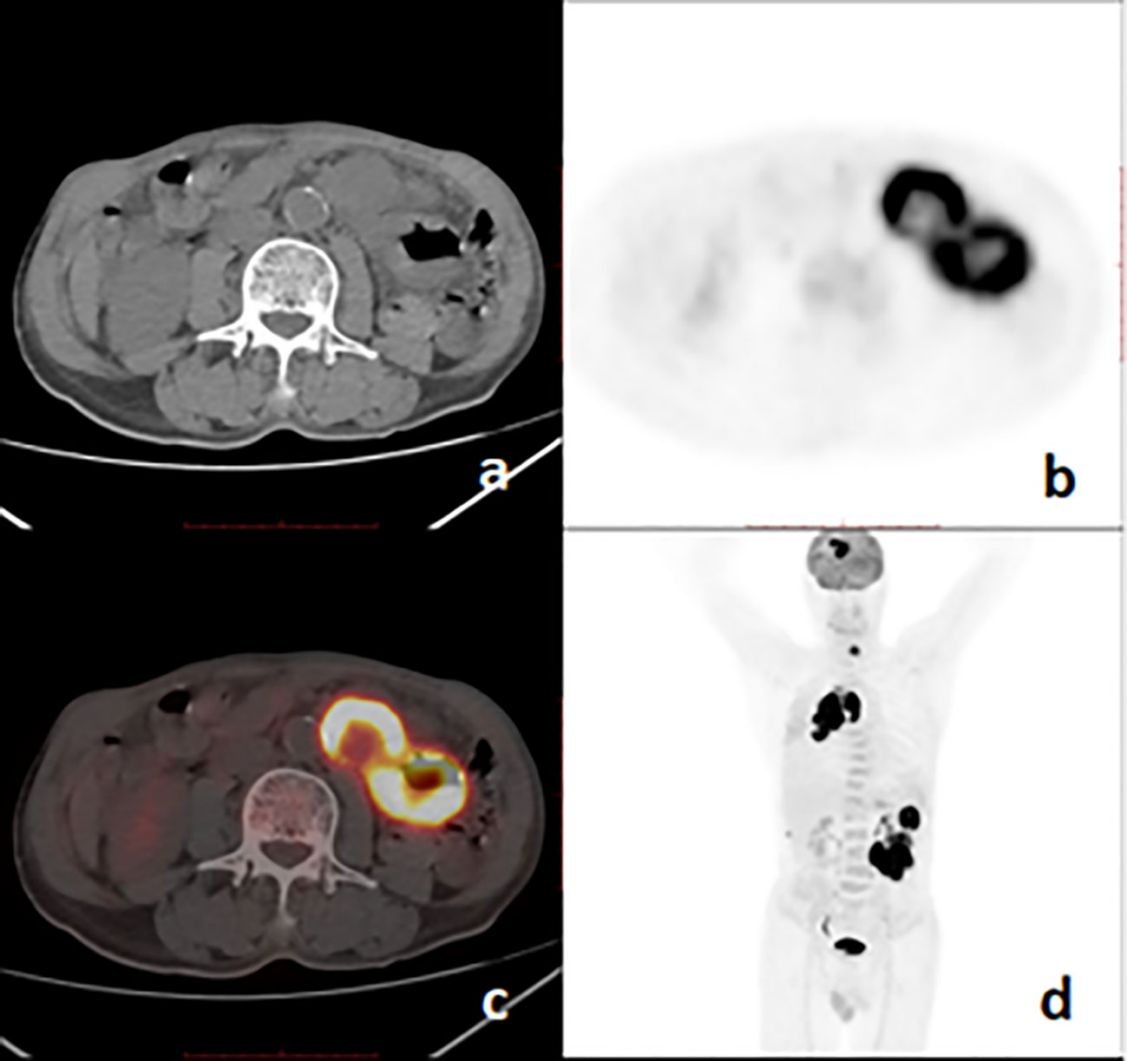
^18^F-FDG PET/CT images of a 67-year-old man with pulmonary sarcomatoid carcinoma showing a large mass with brain, bone, and intestinal metastases. (A), axial CT; (B), PET; (C), fusion image; (D), PET MIP.
